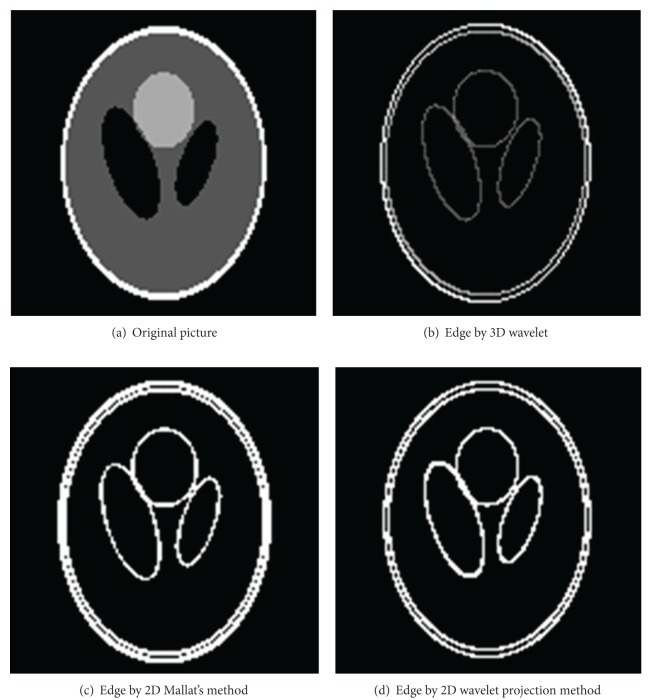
One of 128 Shepp Logan's CT slices and its edge picture.
